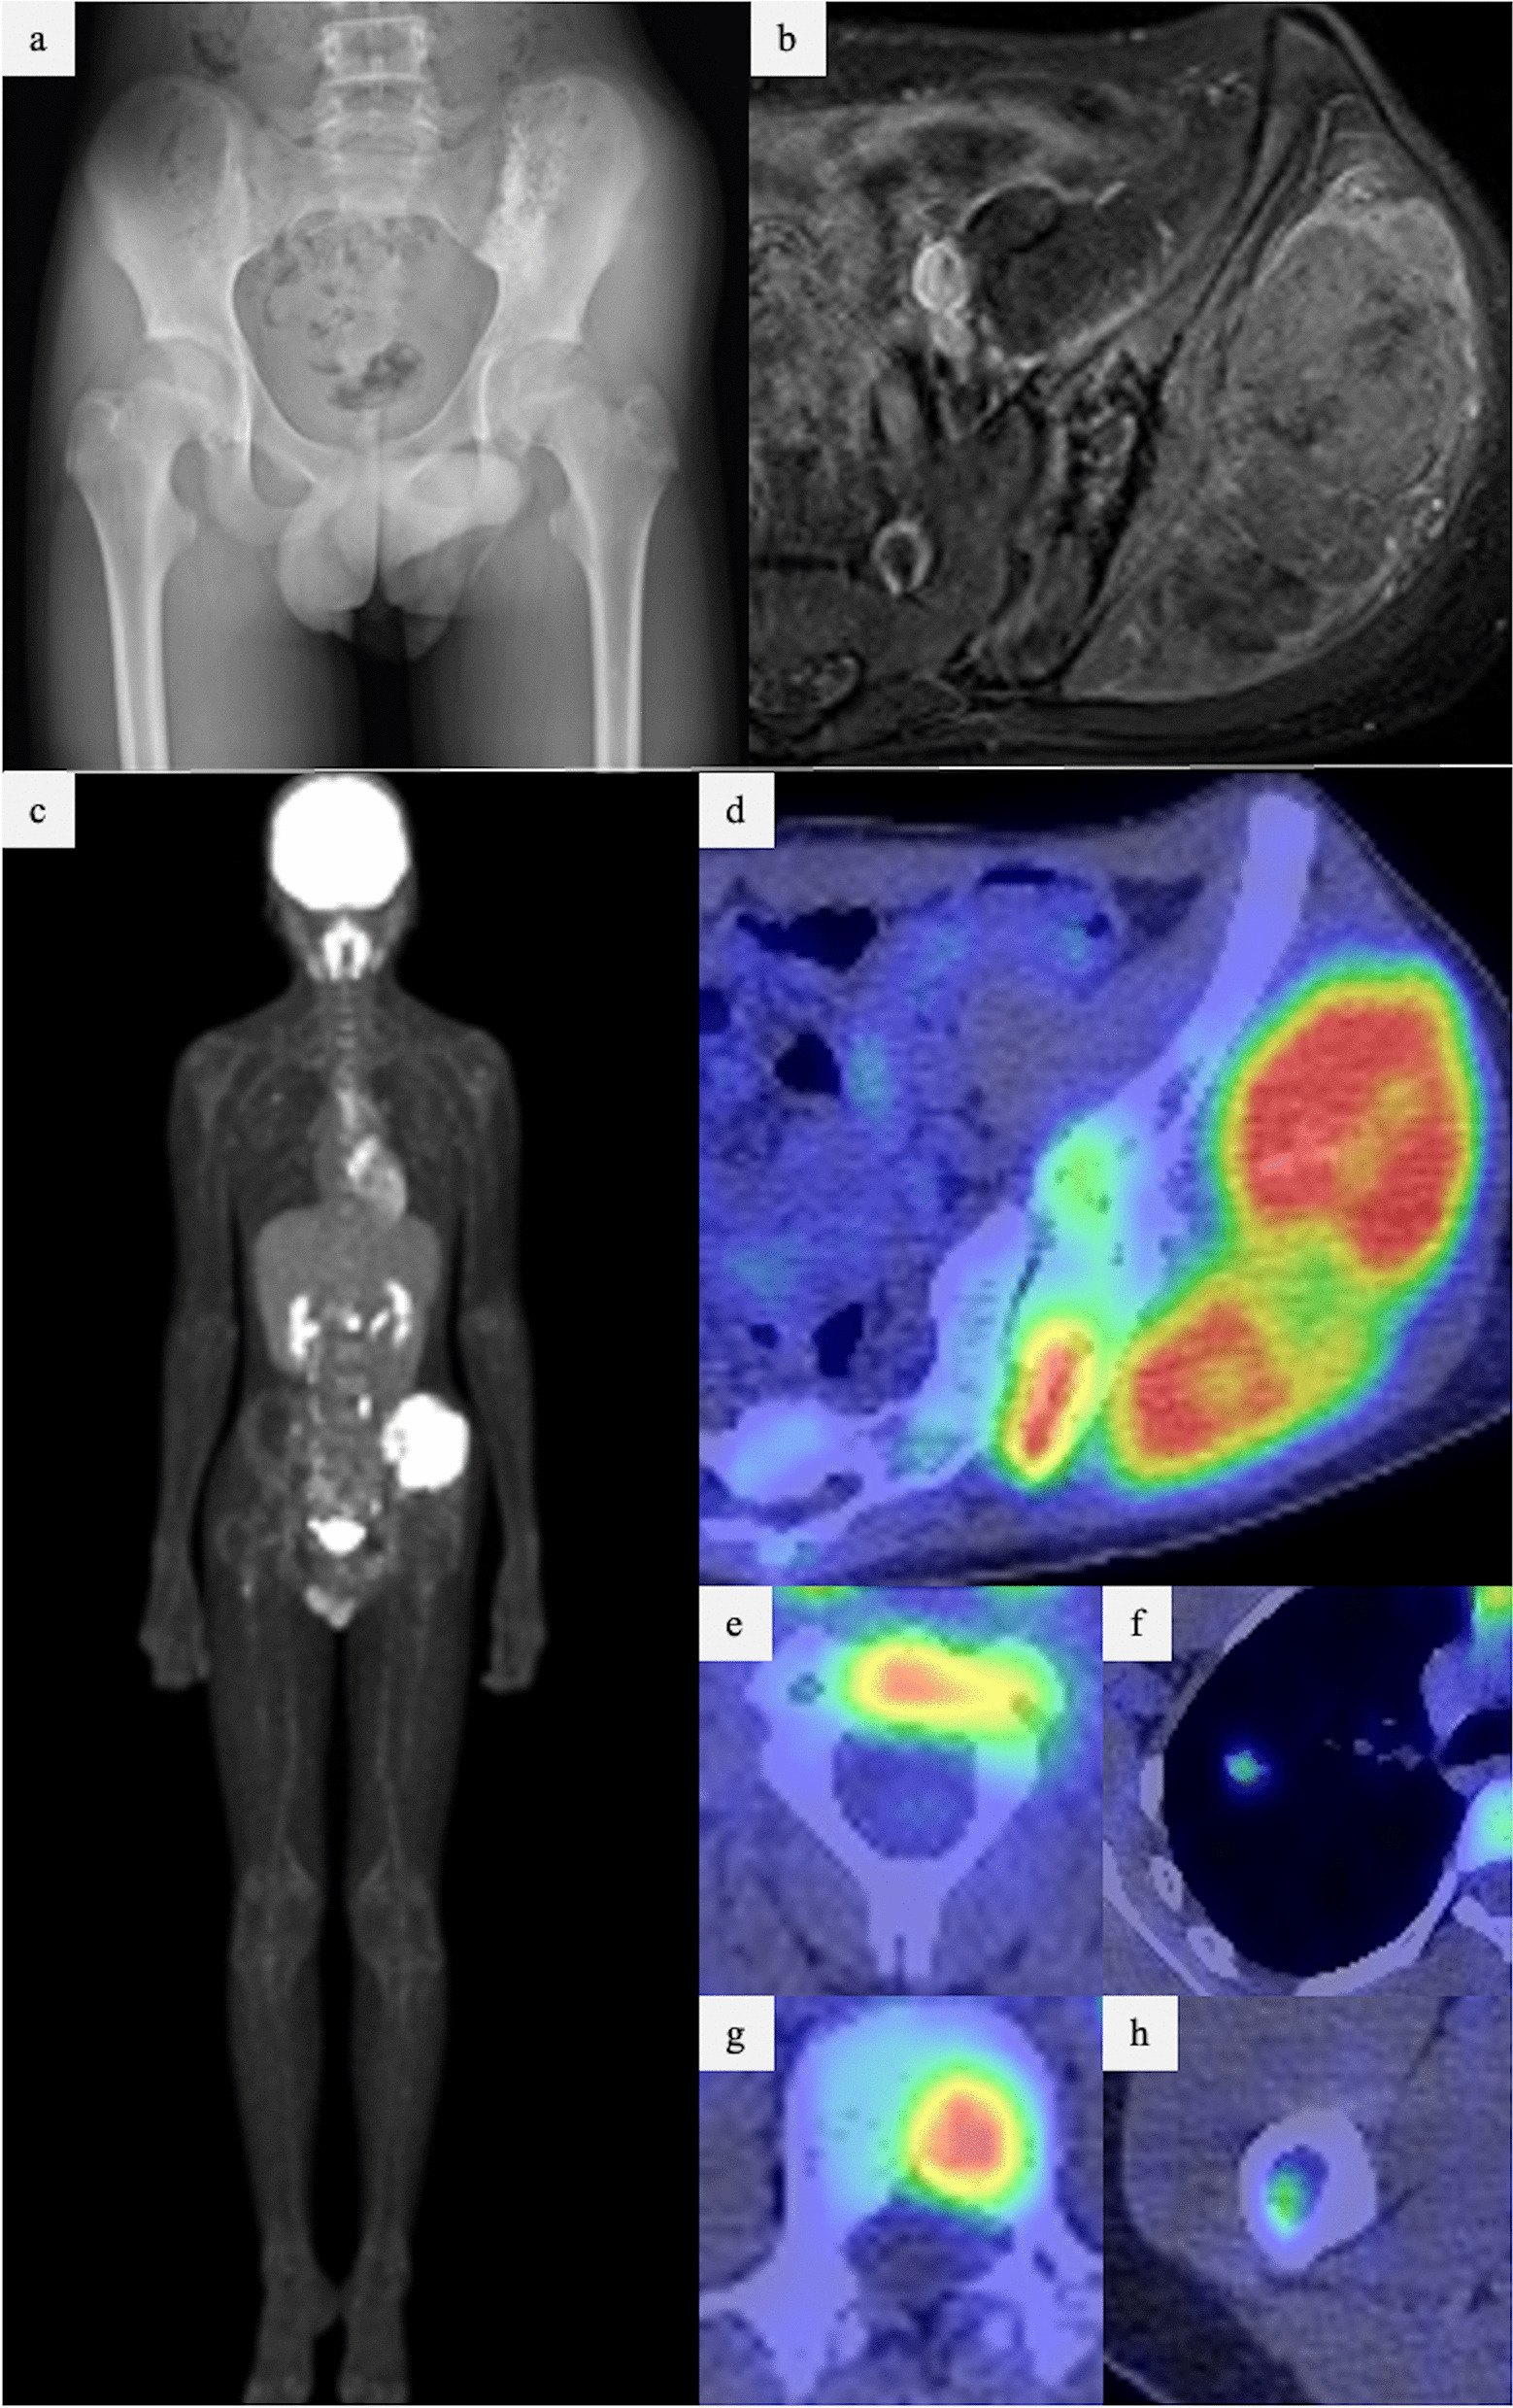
Imaging findings at the first visit to our hospital. a X-ray showing a tumor in the left iliac bone with osteosclerosis and osteolysis. b MRI showing a tumor in the left iliac bone, with a conspicuous extra-bone mass in the gluteus medius muscle. c–h PET–CT showing accumulation in the C2 vertebra (e), right lung (f), L2 vertebra (g), and right femur (h), in addition to the primary tumor (d). CT, computed tomography; MRI, magnetic resonance imaging; PET–CT, positron emission tomography–computed tomography
